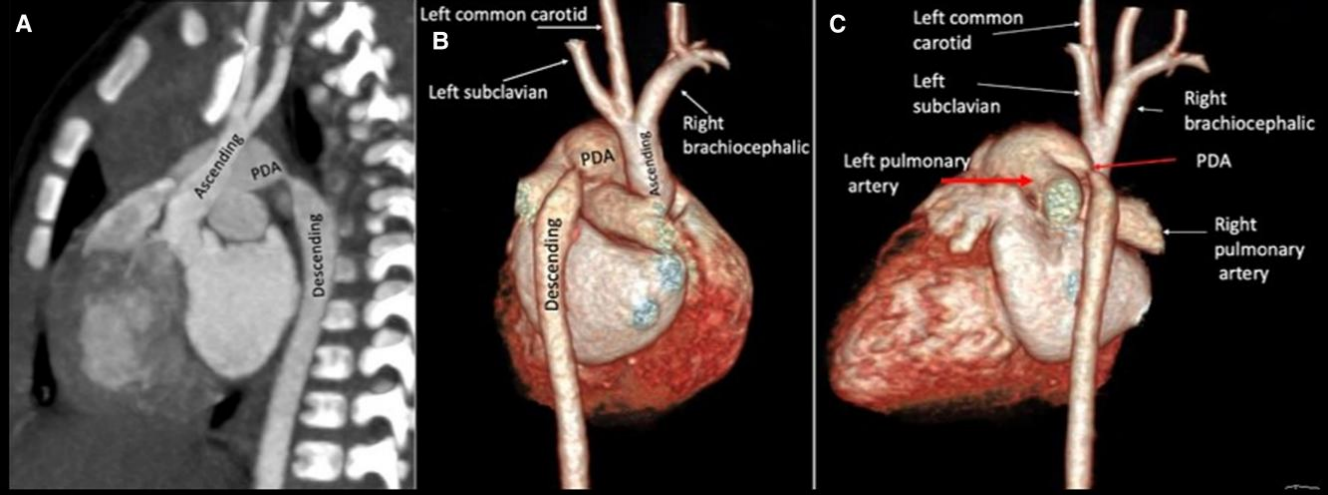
(*A*) Sagittal oblique reformatted image from cardiac computed tomography angiography displays interrupted aortic arch type A. (*B*, *C*) Three-dimensional volume-rendered image shows all three supra-aortic vessels arising from the ascending aorta proximal to the interruption. Patent ductus arteriosus with slight stenosis connects the origin of the left pulmonary artery with the upper descending aorta which lies beyond the interruption.
